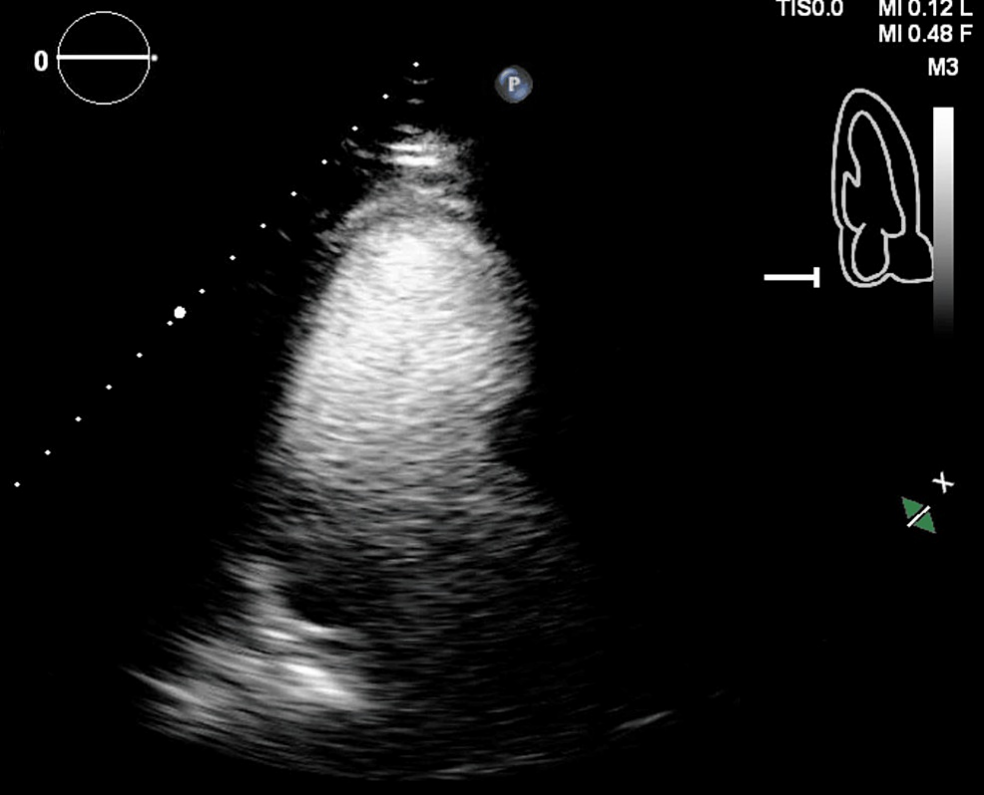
Initial echocardiogram showing apical ballooning.
